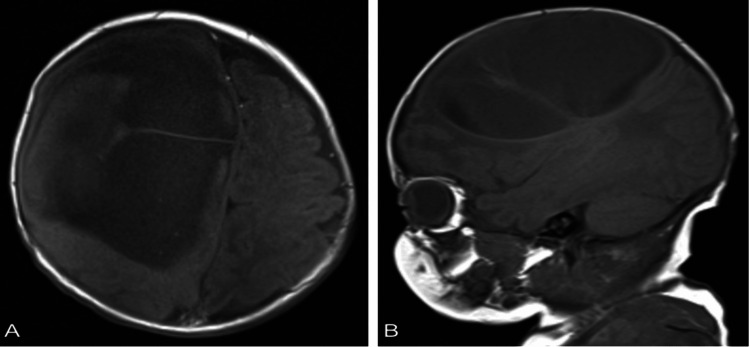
(A) axial T1-weighted MRI (B) sagittal T1-weighted MRI demonstrate a large intraparenchymal cystic mass with low signal intensity, located in the right frontoparietal region of the right cerebral hemisphere.
